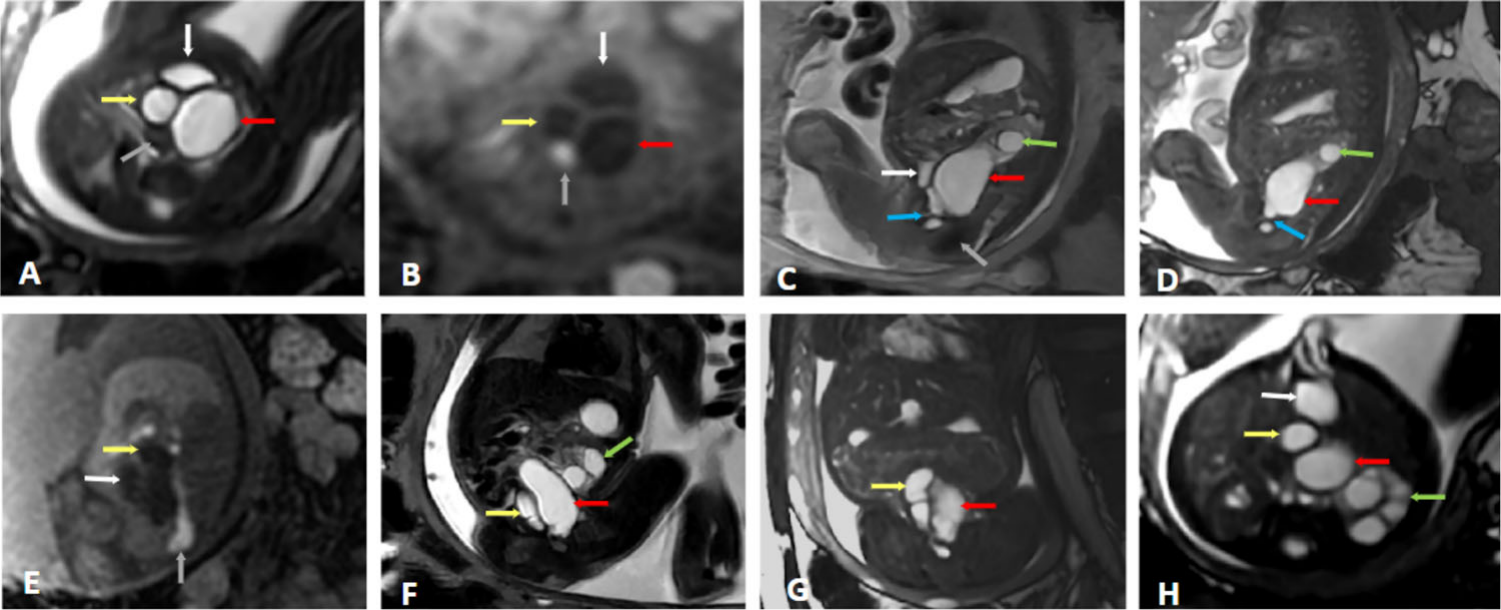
Urogenital sinus and hydrometrocolpos. Gestational age: 27 weeks and 1 day. MRI. A Axial SS FSE T2. B 3D Axial LAVA. C Sagittal SS FSE T2. D Sagittal FIESTA. E 3D Sagittal LAVA. F Coronal SS FSE T2. G Coronal FIESTA. H Axial FIESTA. Two tubular structures with a signal similar to the bladder are identified posterior to the bladder (white arrows) on both sides of the midline, communicating with the upper third of the vagina, corresponding to a didelphys uterus. The right hemi-uterus is located para-medially on the right, measuring 25.4 mm in the cranio–caudal axis (yellow arrows), and the left hemi-uterus, which is larger, is situated para-sagittally on the left, measuring 39 mm in the cranio–caudal axis (red arrows). Additionally, a transverse vaginal septum (blue arrows) is observed at the junction of the upper and middle third of the vagina, measuring 4.2 mm in the anteroposterior axis and 2.2 mm in the cranio–caudal axis, which causes vaginal obstruction. There is no communication with the rectum (gray arrows). The left kidney is dysplastic (orange arrows)
